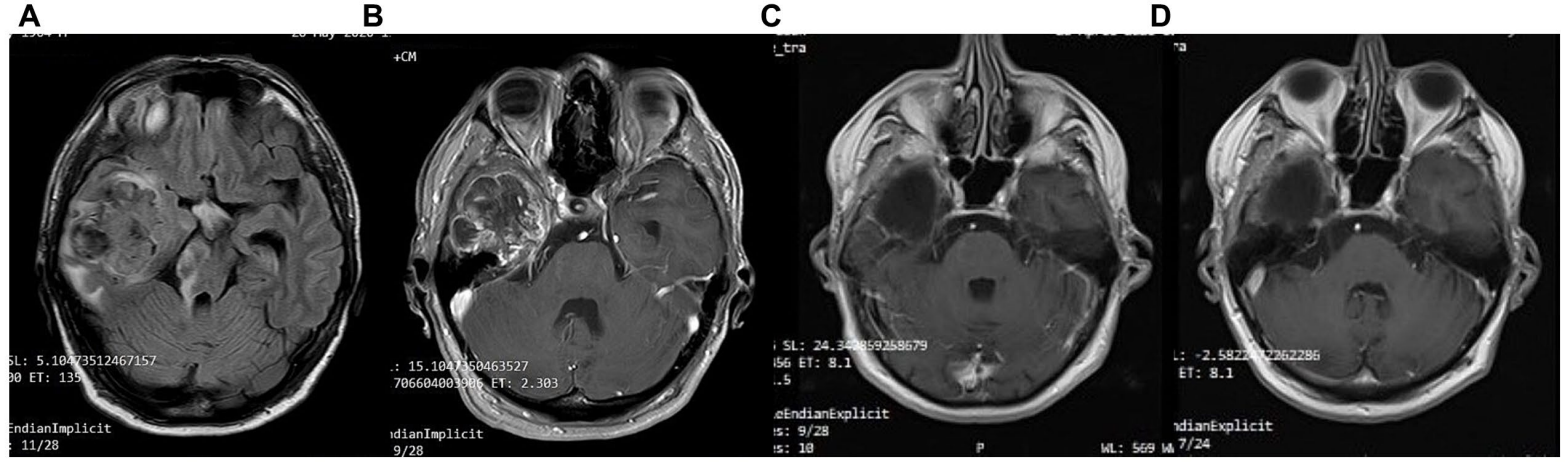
Patient 2: (A) pre-operative brain MRI (T2/FLAIR) (B) pre-operative brain MRI (T1 with contrast) (C) 20-month follow-up brain MRI (T1 with contrast) (D) 40-month follow-up brain MRI (T1 with contrast).
